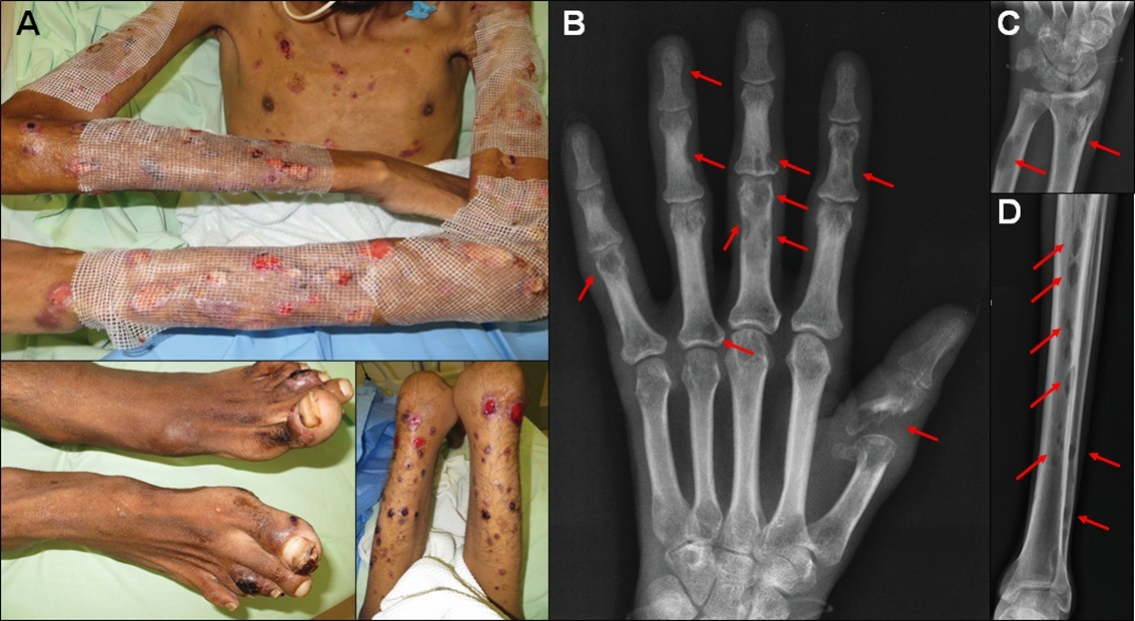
Patient infected with HIV, multifocal bone sporotrichosis (case 6). A) Patient with disseminated sporotrichosis and advanced AIDS. Multiple pleomorphic ulcerated lesions. Radiographs—B) Multiple lytic lesions with destruction of the proximal phalanx of the first right finger. C) Lytic lesions in the right wrist. D) Lytic lesions along the left tibia and fibula. The lesions are pointed by the red arrows. (Source: A—Laboratory of Clinical Research in Infectious Dermatology; B-D—Service of Image of the INI).
